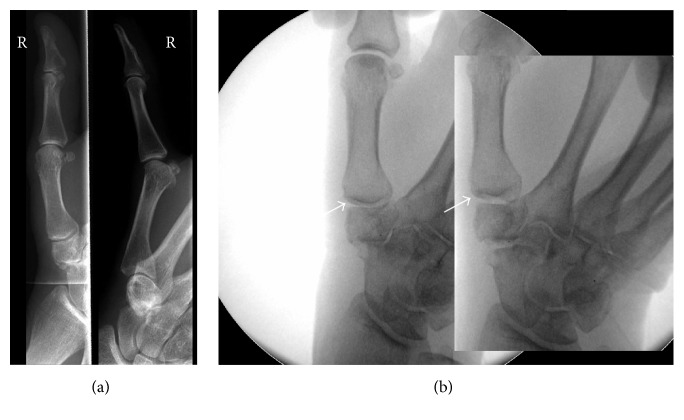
(a) Anteroposterior and lateral X-rays of the right TMC joint of a 71-year-old female with OA of the TMC and scaphotrapeziotrapezoid (STT) joints classified as Eaton and Littler III. The patient reported pain since 71 weeks and had undergone anti-inflammatory pain medication as needed since then. (b) Fluoroscopic images of the right hand before and after PRP injection resulting in TMC and STT joint distension due to intra-articular PRP application.
